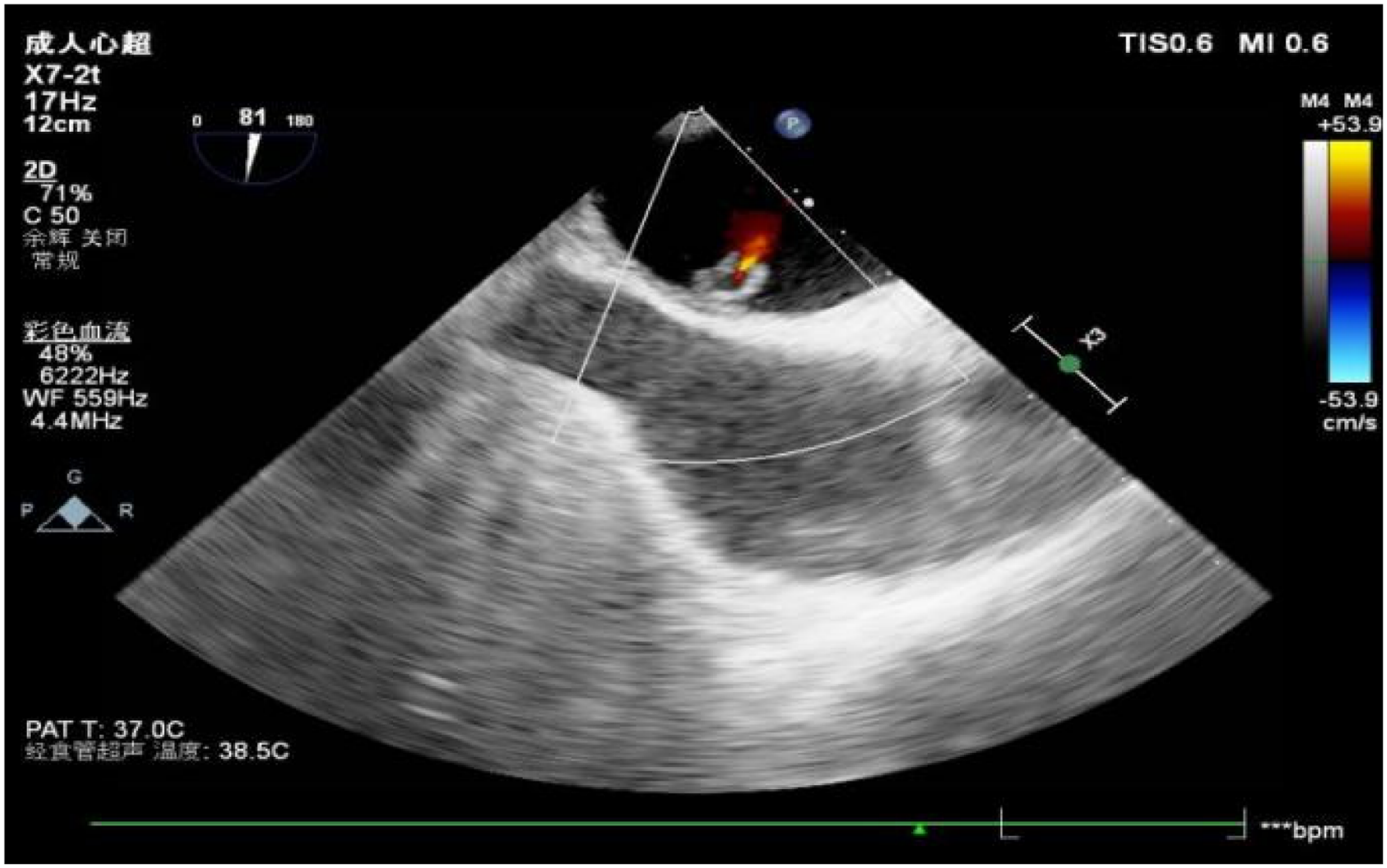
Color Doppler images showed intra-tumoral blood flow and blood stream draining from the mass.
